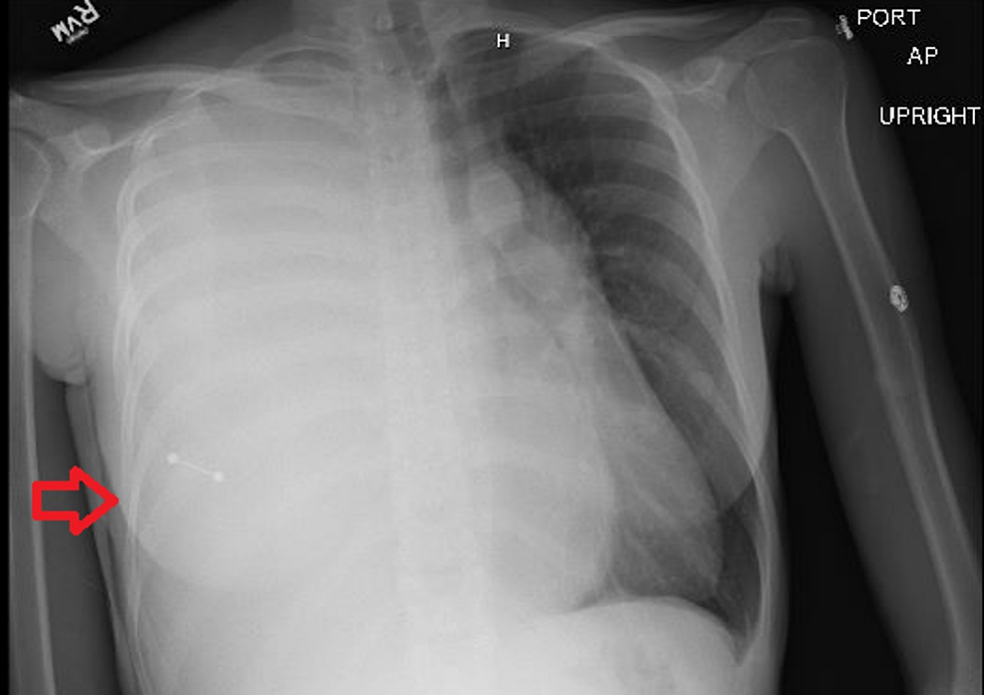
CXR on admission day showed complete opacification of the right side (red arrow) with a left-sided tracheal deviation AP: anterior-posterior, CXR: chest X-ray, H: hilum and mediastinum, Rvm: Relevance Vector Machine.
